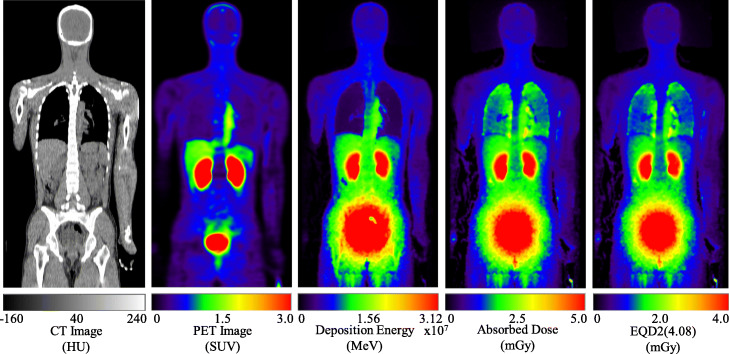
Representative CT and PET images (90 min average) of a volunteer after the injection of NKO-035 labeled with ^18^F and the corresponding deposition energy, absorbed dose, and EQD2(4.08) maps obtained from RT-PHITS
